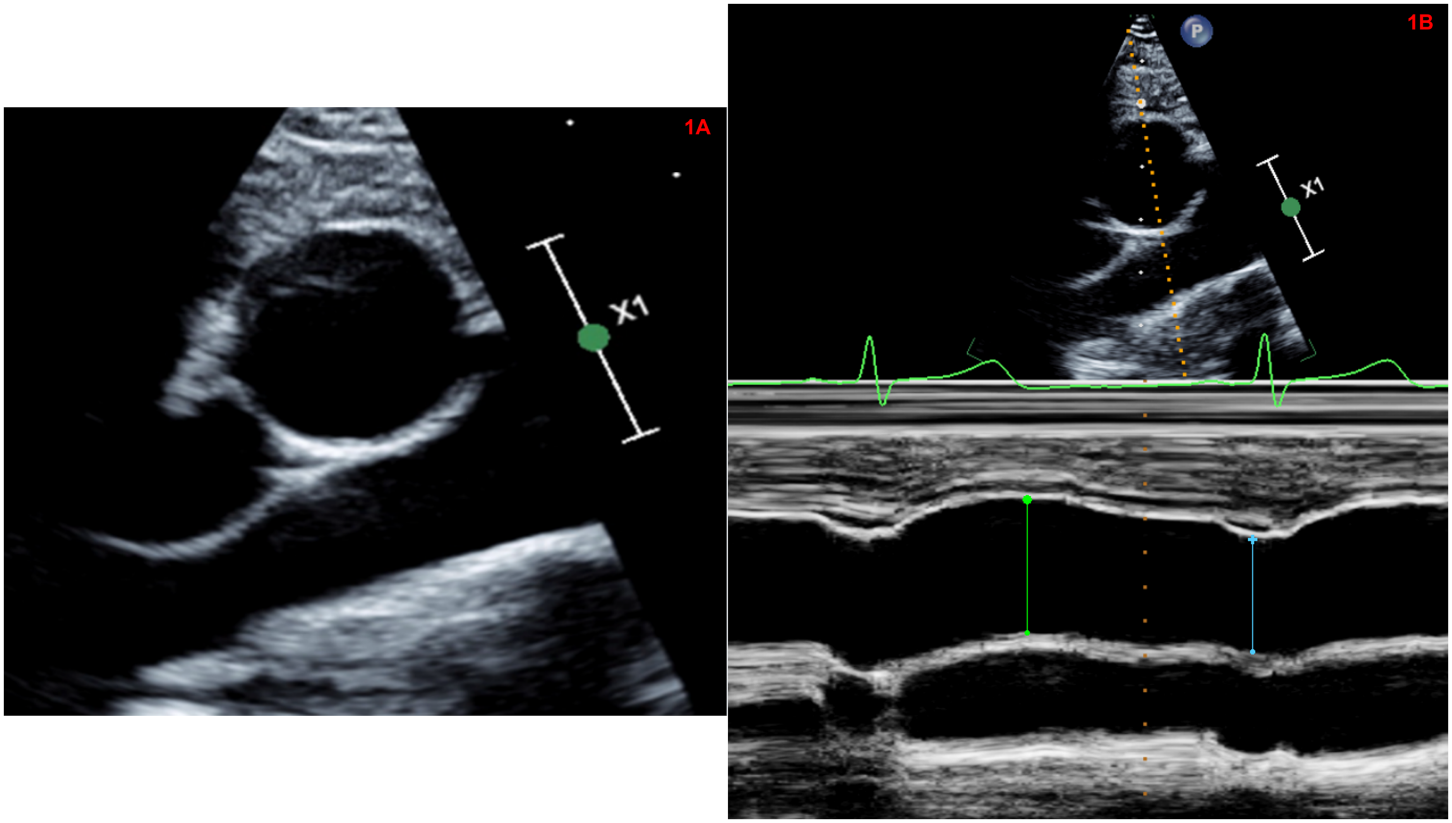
(A) Two-dimensional high right parasternal short axis view of the ascending aorta (at the level of the right pulmonary artery). (B) Two-dimensional guided M-mode of the ascending aorta from high right parasternal short axis view.
